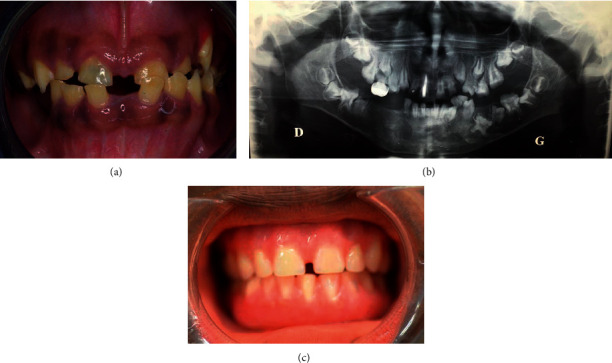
Clinical and radiological views of the proband. (a) Intraoral photograph of the patient showing hypocalcified enamel and eruption anomalies. The enamel is dark yellow, streaked, and severely worn out. The proband's teeth are in the transitional dentition phase, with major diastemas. (b) Panoramic radiograph of the proband showing retained, impacted, and missing teeth; generally thin or absent enamel; taurodontism on first permanent molars; one temporary molar restored with a preformed metallic crown; and the central right incisor endodontically treated. (c) Intraoral photograph immediately after prosthetic rehabilitation of the patient with a complete removable denture. An anterior disastema is preserved for esthetics.
